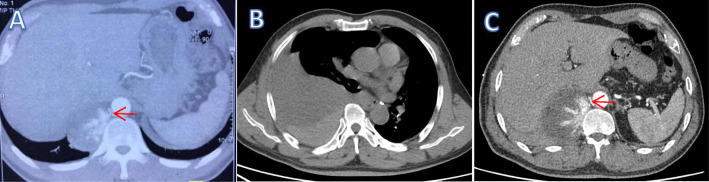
a. Chest CT 3 years prior showed a mass between the right spine and diaphragm. b. Chest CT showed right massive hemothorax. c. Chest CT showed growing mass with distinct feeding vessel
